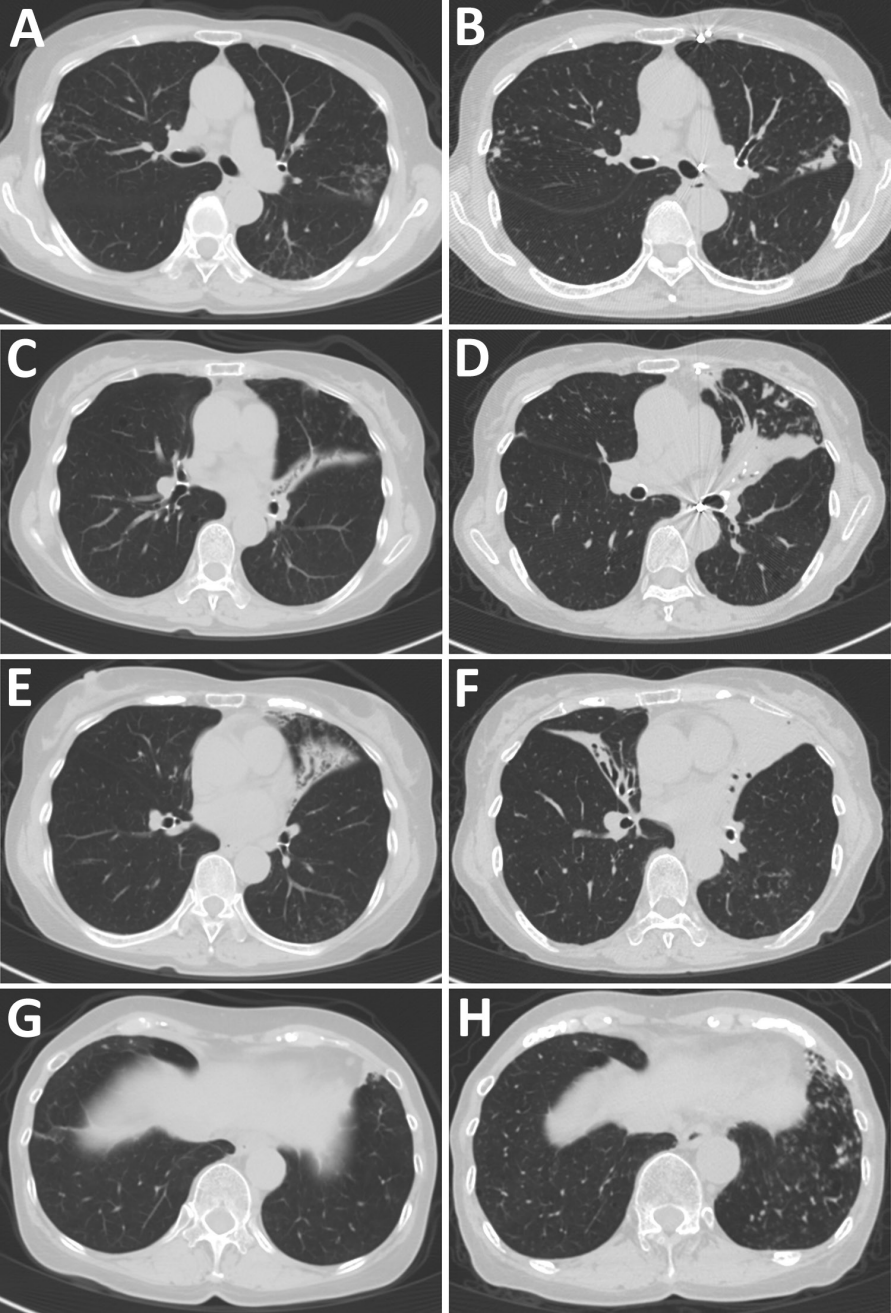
Comparison of chest computed tomography findings over time for patient who had chronic pulmonary disease caused by *Tsukamurella toyonakaense*. Findings are shown from before *Tsukamurella* species was detected (A, C, E, and G) and 6 years later (B, D, F, and H). A and B show that nodules in right segment 2 and left segment 6 were unchanged. C and D show that bronchiectasis in lingula had progressed. E and F show that bronchiectasis newly appeared in the middle lobe. G and H show that nodules newly appeared in left segments 8–10.
